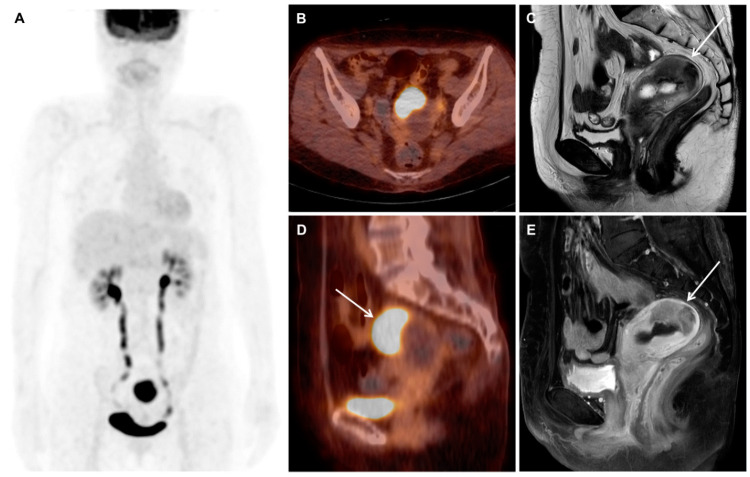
A 67-year-old postmenopausal woman who presented with vaginal bleeding underwent hysteroscopy, which revealed a protruding mass in the uterine cavity. Hysteroscopic resection was done and pathologic examination suggested a diagnosis of endometrioid adenocarcinoma with FIGO grade I. Carbohydrate antigen 125 (CA 125) was within the normal range (15.5 U/mL). She underwent an ^18^F-FDG PET/CT scan and a pelvic MRI scan for tumor staging. The ^18^F-FDG PET/CT images (A, B, D; maximum intensity projection image, transaxial and sagittal fused PET/CT images) showed an intense hypermetabolic mass (arrow, SUVmax of 23.1) in the uterine fundus and no evidence of metastasis. The MRI images (C, E; T2-weighted image and gadolinium-enhanced T1-weighted image) showed a mass in the uterine fundus, which was confined to the myometrium (arrows). Since the epicenter of the tumor was in the myometrium, leiomyosarcoma rather than endometrial cancer was suspected. As there was no evidence of distant metastasis, she underwent hysterectomy with bilateral salpingo-oophorectomy and pelvic lymph node dissection.
